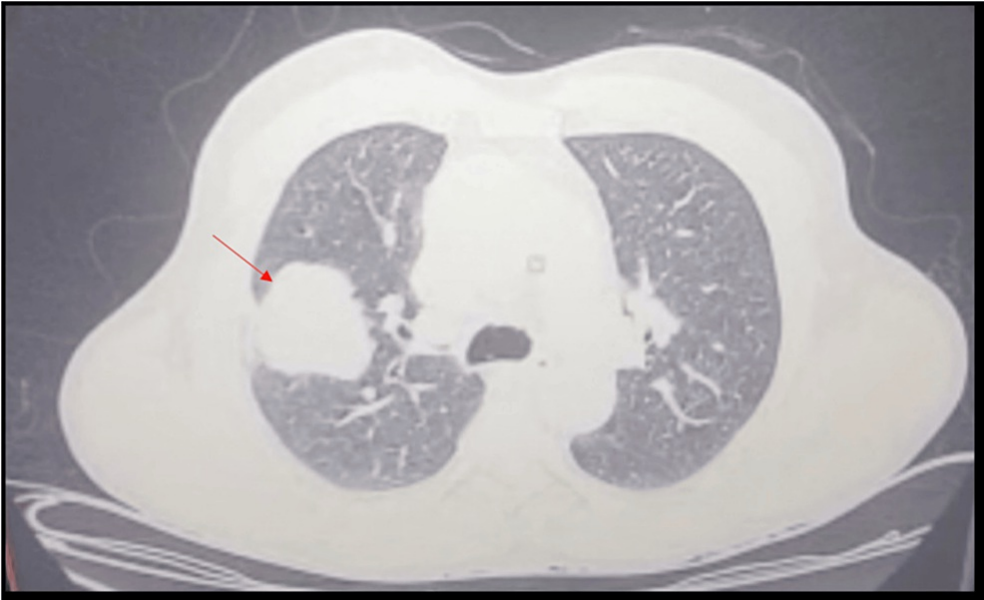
Chest HRCT (lung window)
